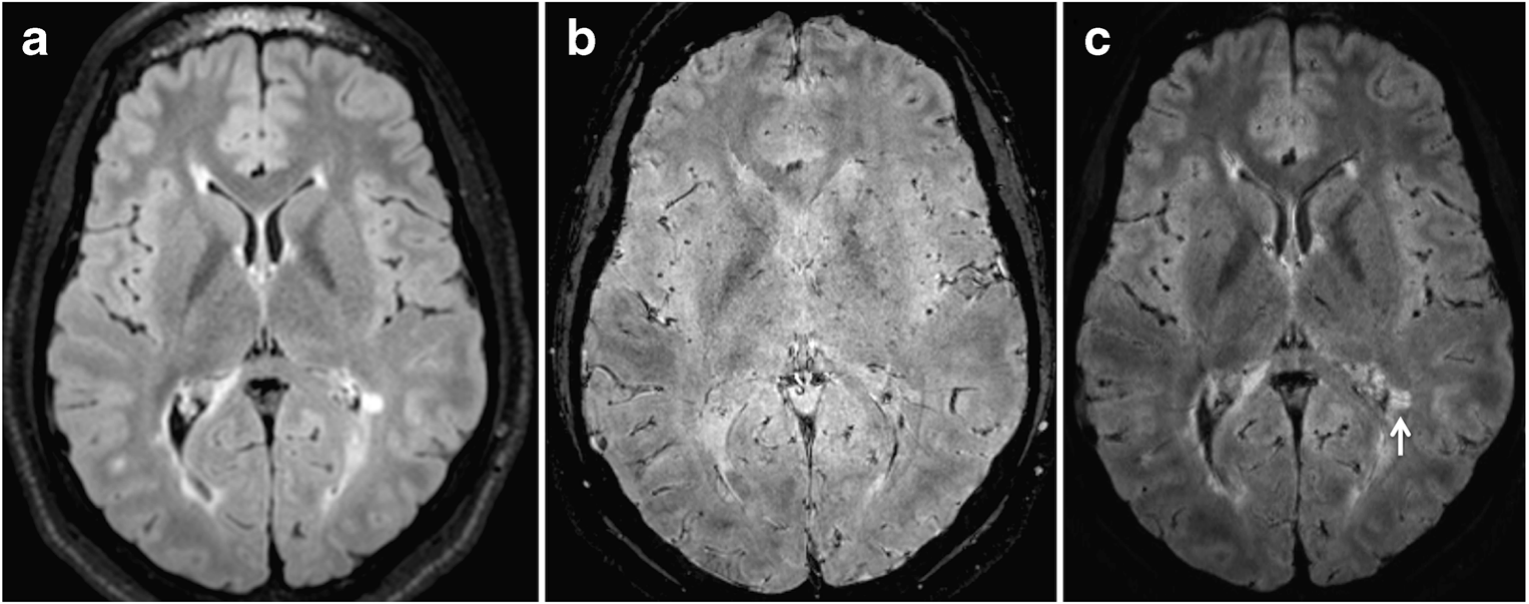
Construction of FLAIR* images. (a) FLAIR, axial slice. (b) T_2_*, axial slice. (c) Combined images create FLAIR*, axial slice. White arrow indicates intralesional vein
